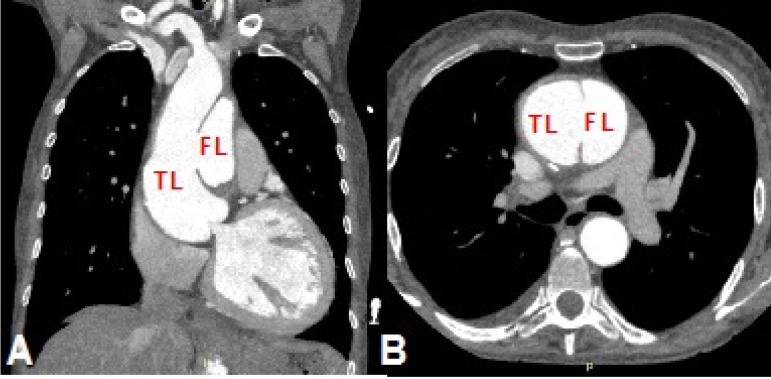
Case 6: computed tomography (CT) angiography of aortic dissection
